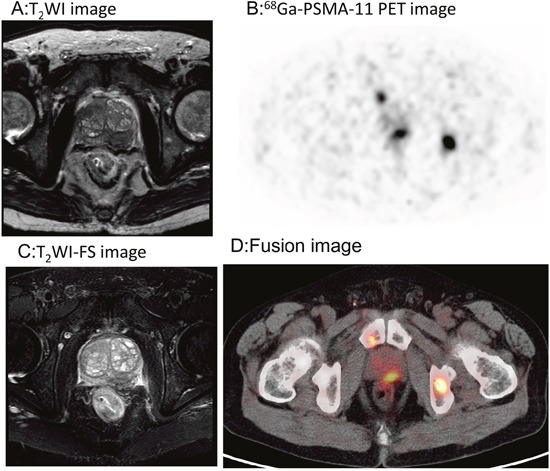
68Ga-PSMA-11PET/CT versus MRI for the evaluation of the primary prostatic lesions Focal uptake was found in the left peripheral zone of the prostate gland, which matched the hypointensity signal on MRI. A. T_2_WI image. B. ^68^Ga-PSMA-11 PET transverse image. C. T_2_WI-FS image. D. Fusion image.
